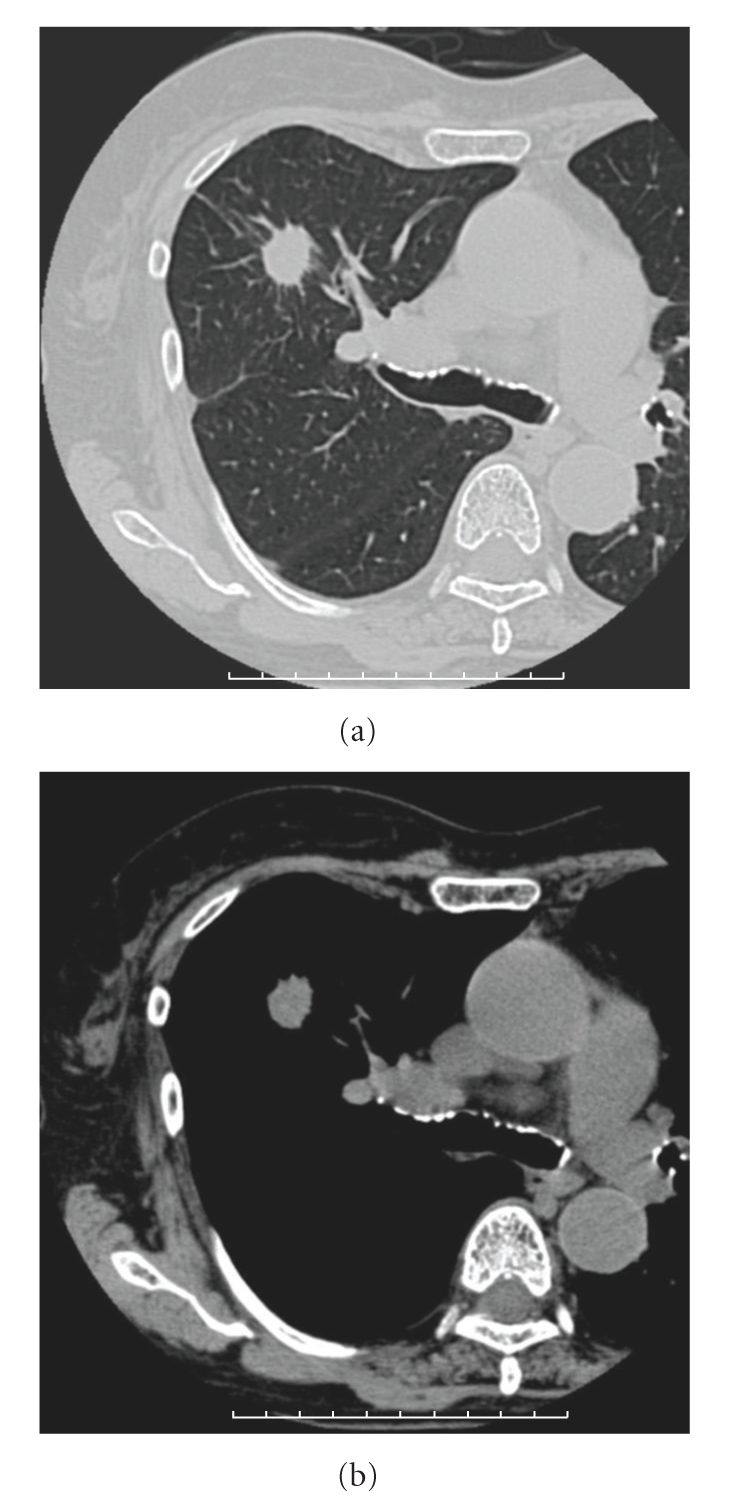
CT images before SBRT showing a solitary lung tumor in the right upper lobe.
